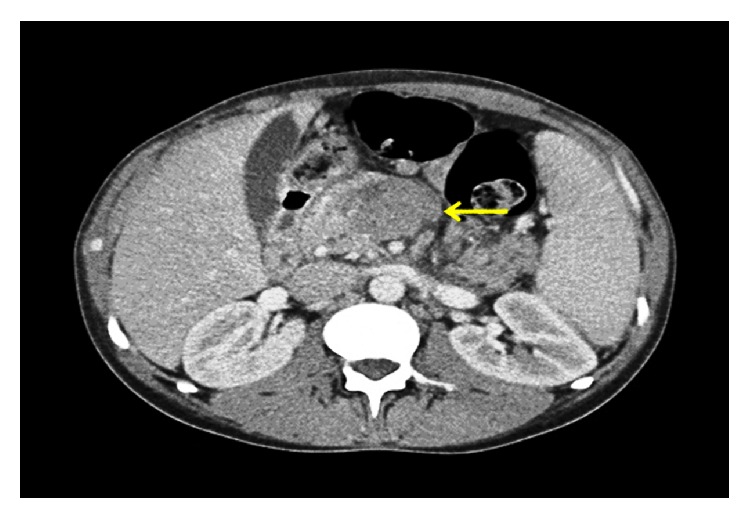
Contrast-enhanced computed tomography at the level of pancreas. Arrow demarcates multiple peripancreatic soft tissue masses to left of the uncinate process.
